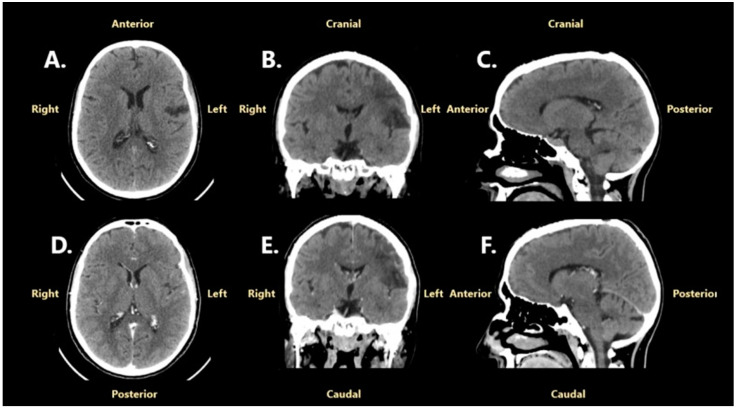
A cranial CT scan performed in December 2023, 3 months after the neurosurgical intervention: (A)—non-contrast, axial projection; (B)—contrast enhancement, coronal projection; (C)—non-contrast, sagittal projection; (D)—contrast enhancement, axial projection; (E)—contrast enhancement, coronal reconstruction; (F) contrast enhancement, sagittal reconstruction.
